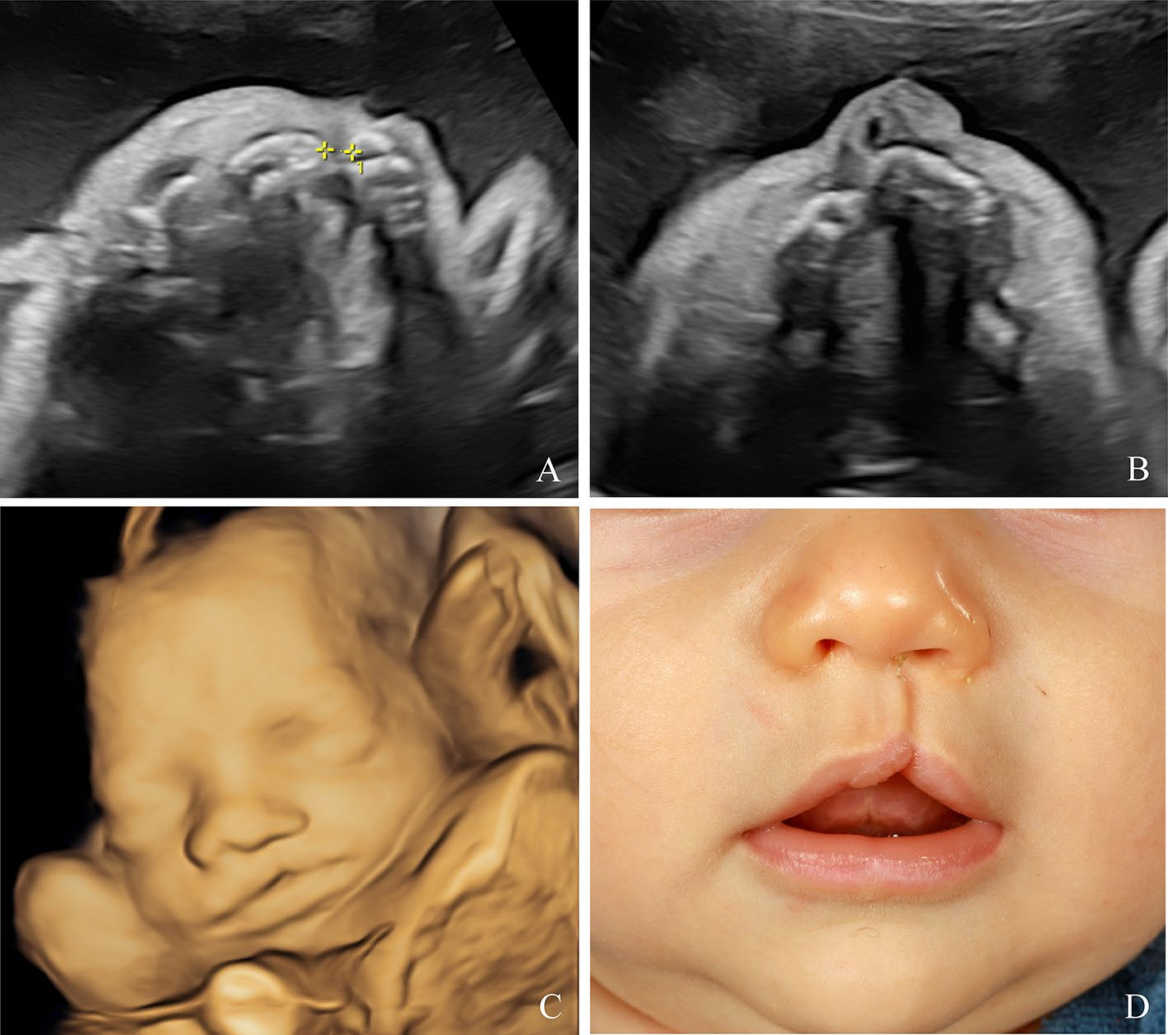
Unilateral cleft lip and palate, visualized on ultrasound during the prenatal period in 2D, in transverse sections (A, B), and in 3D (C). Postnatal finding (D)
